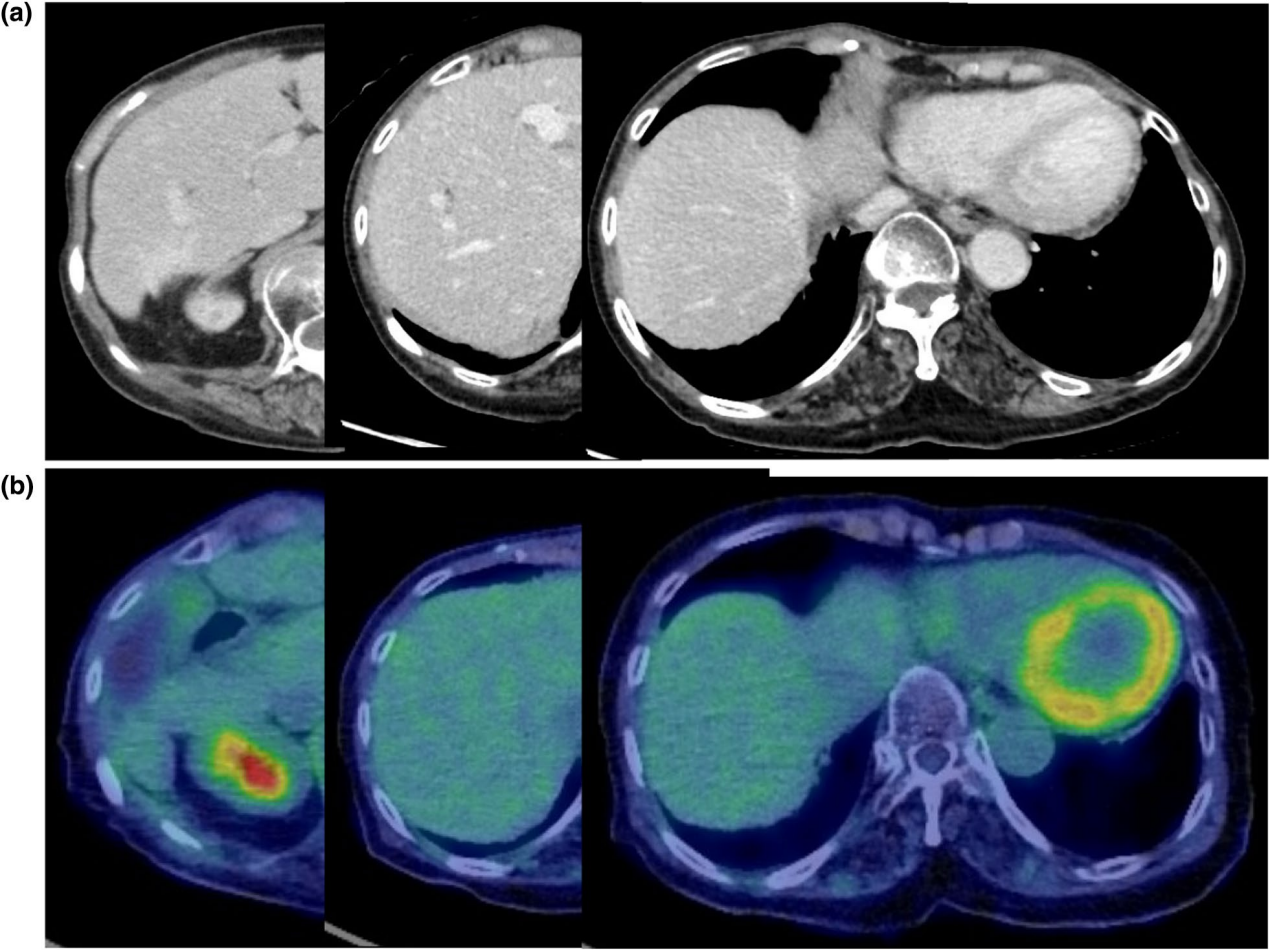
a Abdominal CT showing complete remission of liver metastases. *CT* computed tomography. b PET–CT scan showing no sign of recurrence. *PET–CT* positron emission tomography–computed tomography
